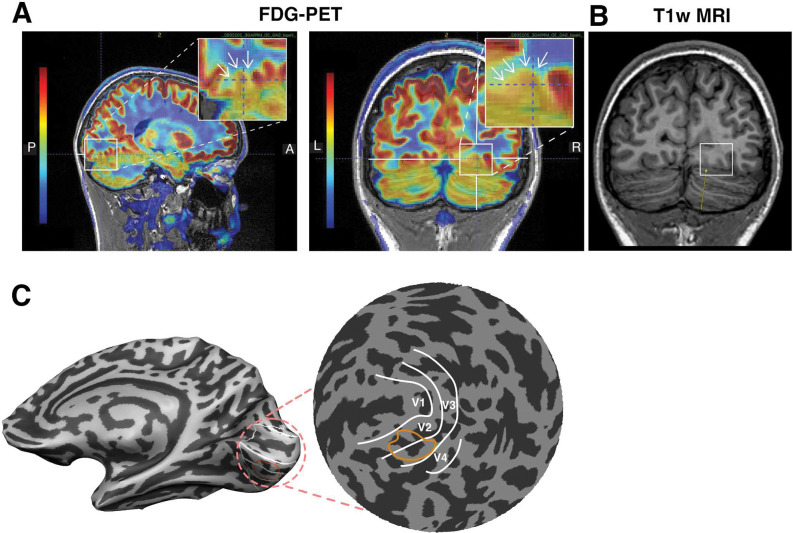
FDG-PET showing reduced glucose metabolism in pRF-defined regions of the right ventral visual cortex. (A) Sagittal and coronal slices of the FDG-PET map. Insets highlight the lingual gyrus, with arrows indicating areas of hypometabolism. (B) The same coronal slice of a T1-weighted image shows cortical gray/white matter blurring (white rectangle). (C) Right hemisphere cortical surface view with a zoomed-in inset of the occipital lobe. White lines delineate visual field map boundaries, and the orange outline indicates the hypometabolism on the cortical surface.
